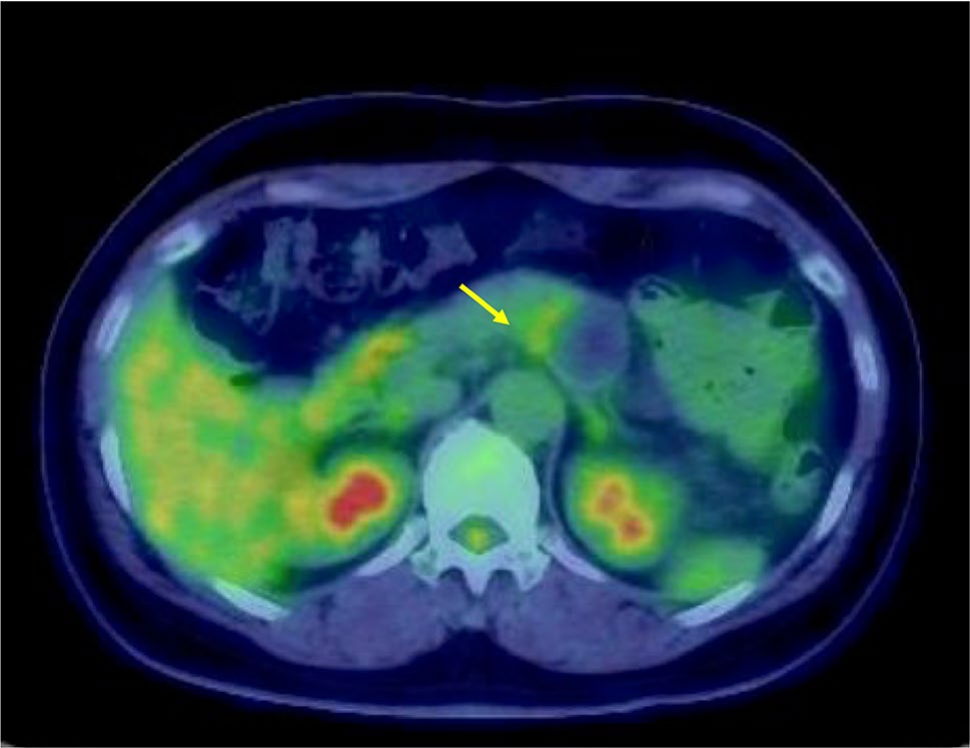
^18^F-fluorodeoxyglucose (FDG)-positron emission tomography combined with a computed tomography scan showed mild ^18^F-FDG accumulation in the papillary side of the cystic lesion (maximum standardized uptake value, 3.5) (yellow arrow)
